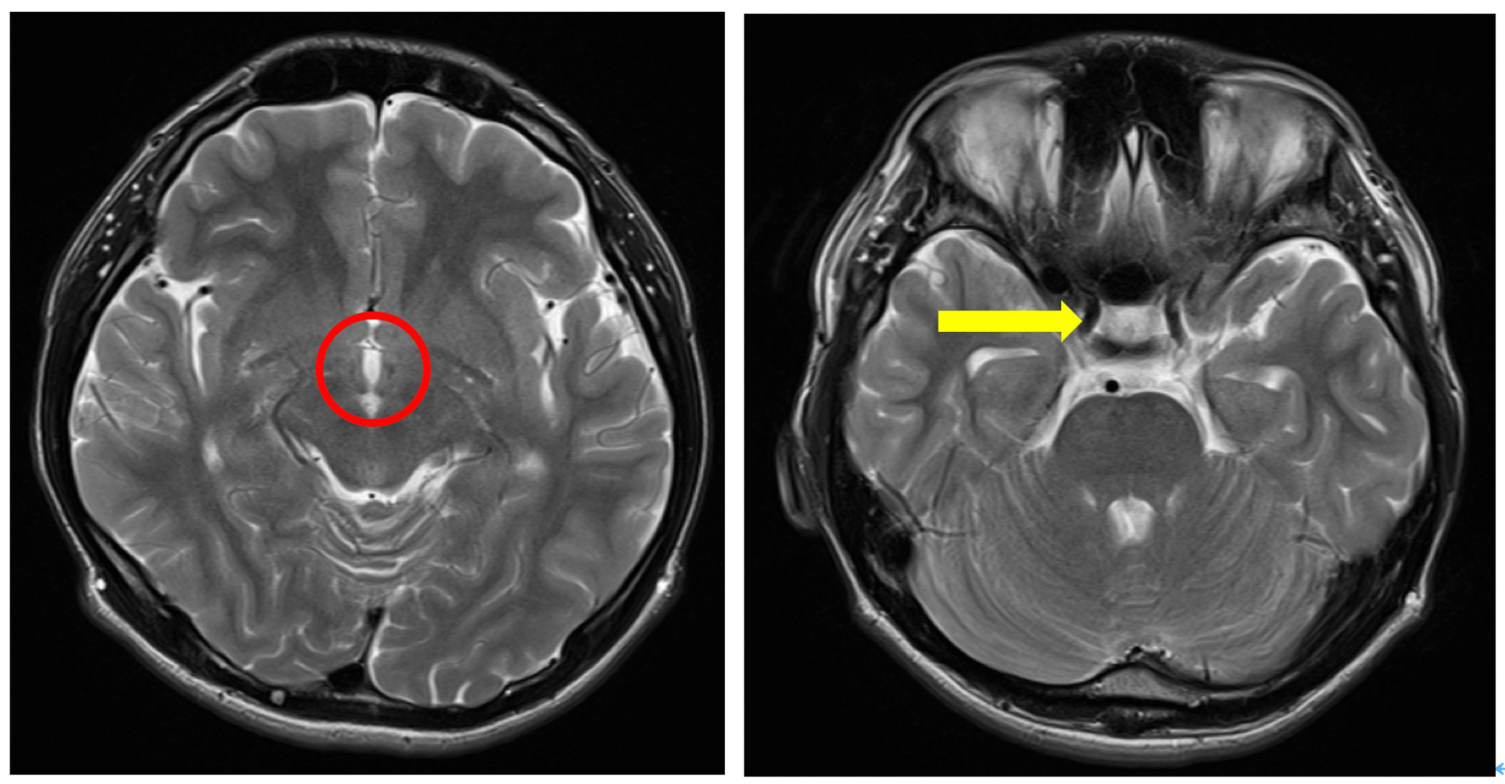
Axial T2-weighted MRI of the hypothalamus and pituitary gland on hospital day 1 The hypothalamus is indicated by a red circle, and the pituitary gland is marked with a yellow arrowhead.
